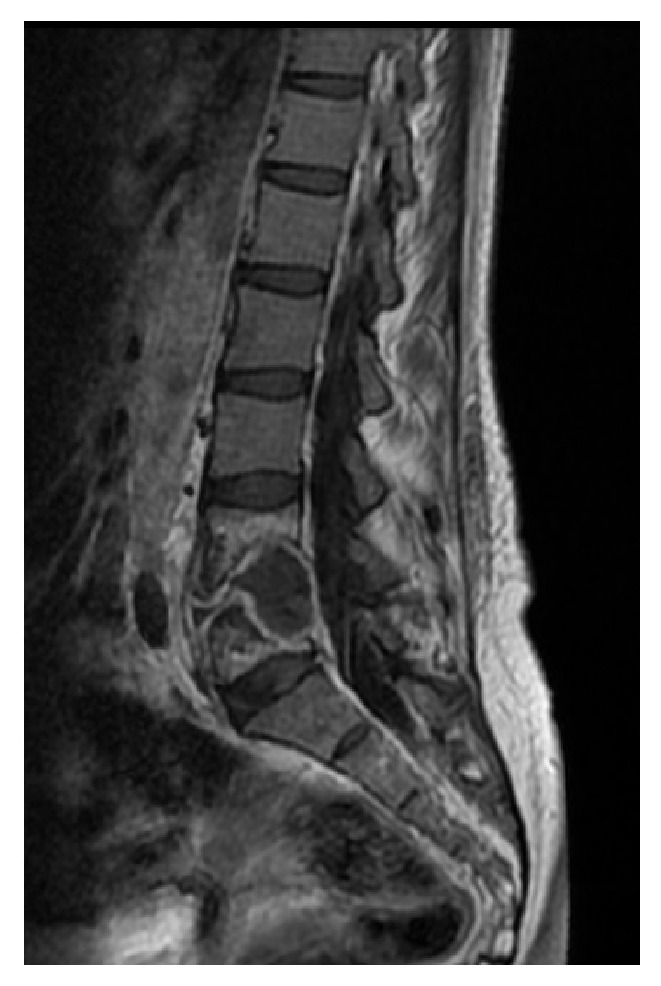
Sagittal view of MRI lumbar spine. T1 flair image with contrast demonstrating diskitis and osteomyelitis with expansile mass extending into epidural space.
